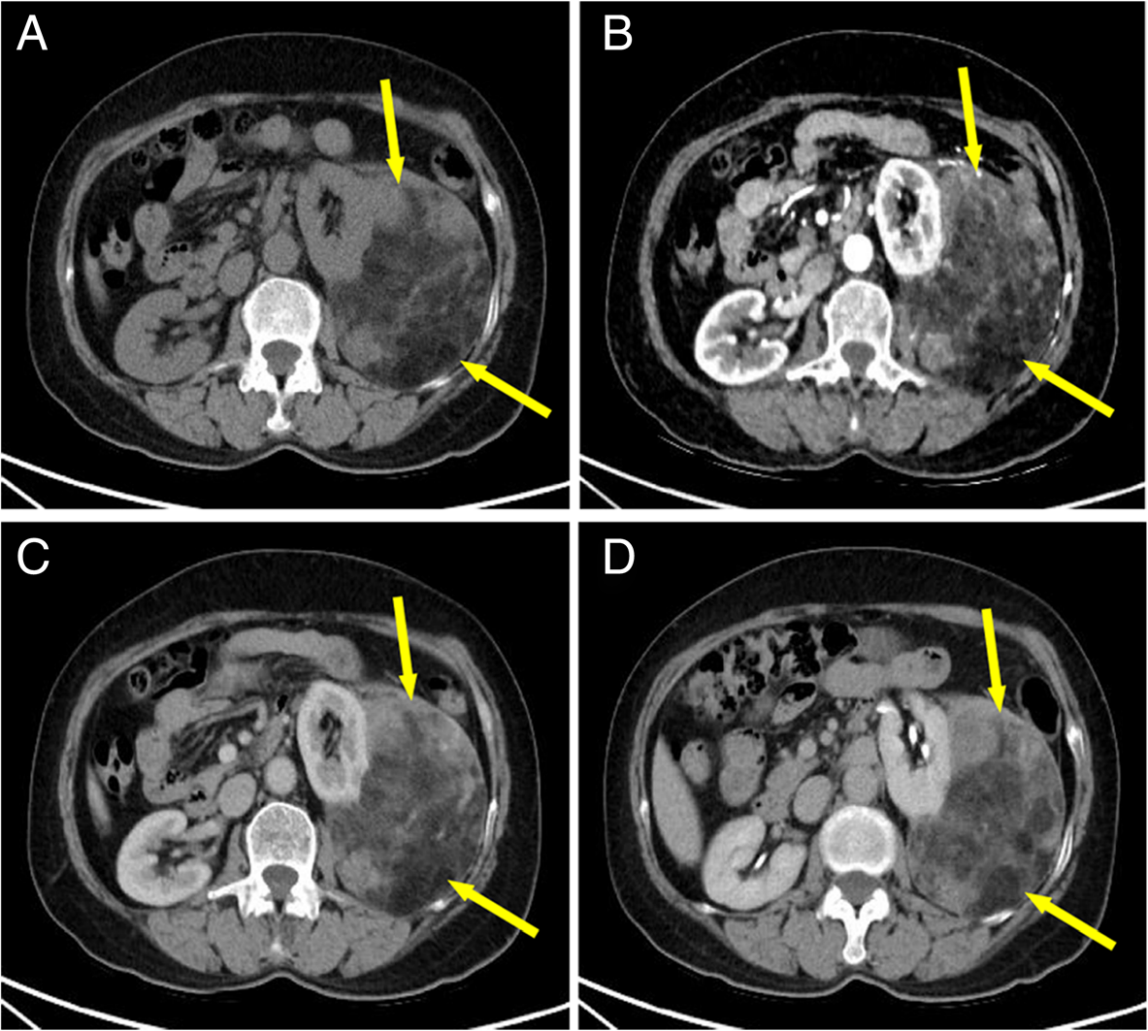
The complex lesion of the kidney on computed tomography (CT). a. Plain CT image showed a large mixed density mass in the left renal fascia, which was closely related to the left kidney (yellow arrows). b. Dynamic contrast CT (arterial phase) showed the mass was obviously uneven, and small vessels could be seen around the lesion (yellow arrows). c. Dynamic contrast CT (venous phase) showed the enhancement degree of the mass decreased compared to that in the arterial phase (yellow arrows). d. Dynamic contrast CT (equilibrium phase) showed the degree of enhancement was similar to that of venous phase (yellow arrows)
